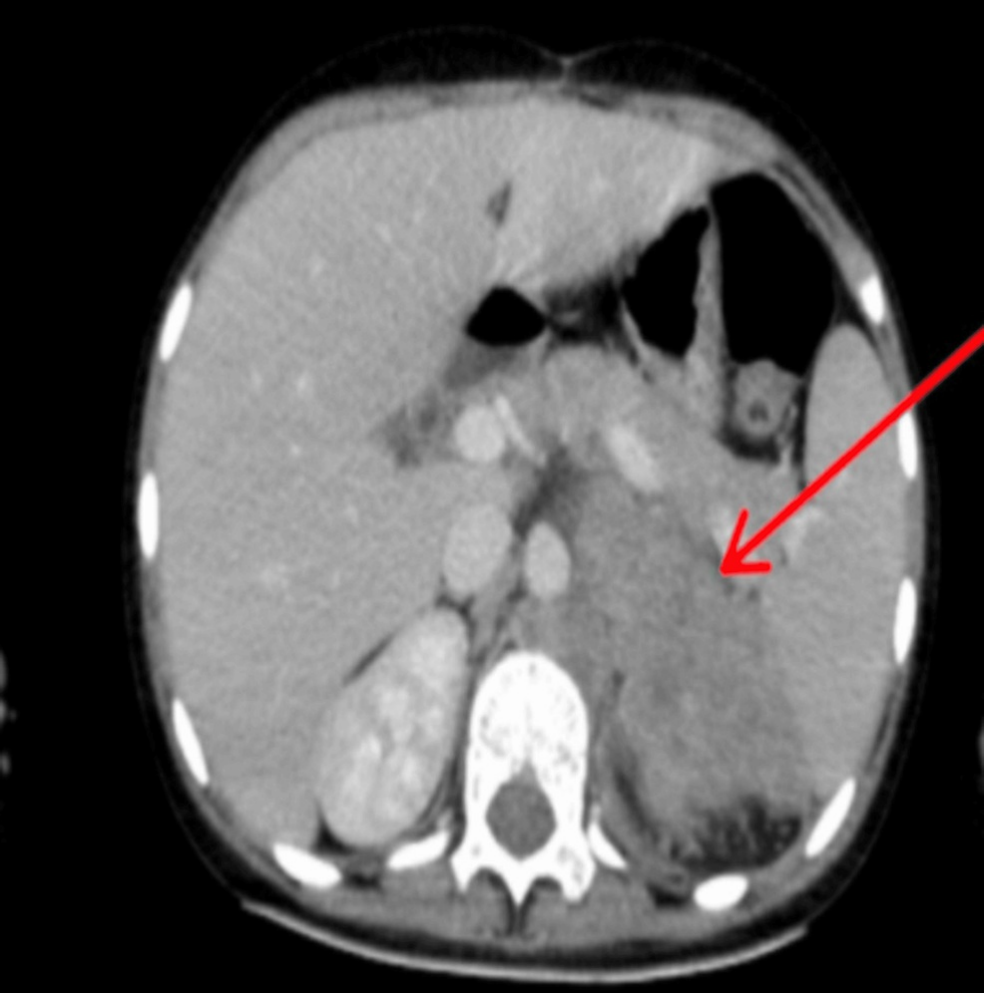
Contrast-enhanced CT showing an enhancing mass of the left adrenal gland with retroperitoneal enhancing lymph nodes noted with a partial aortic abutment.
